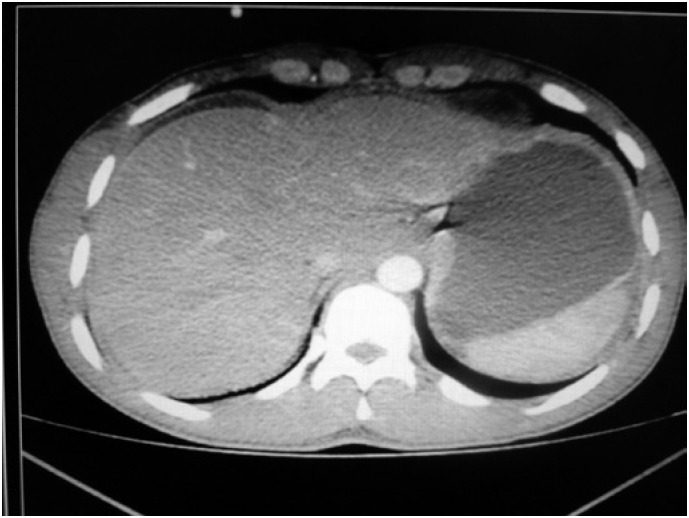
Computed tomography showed a large amount of free fluid
